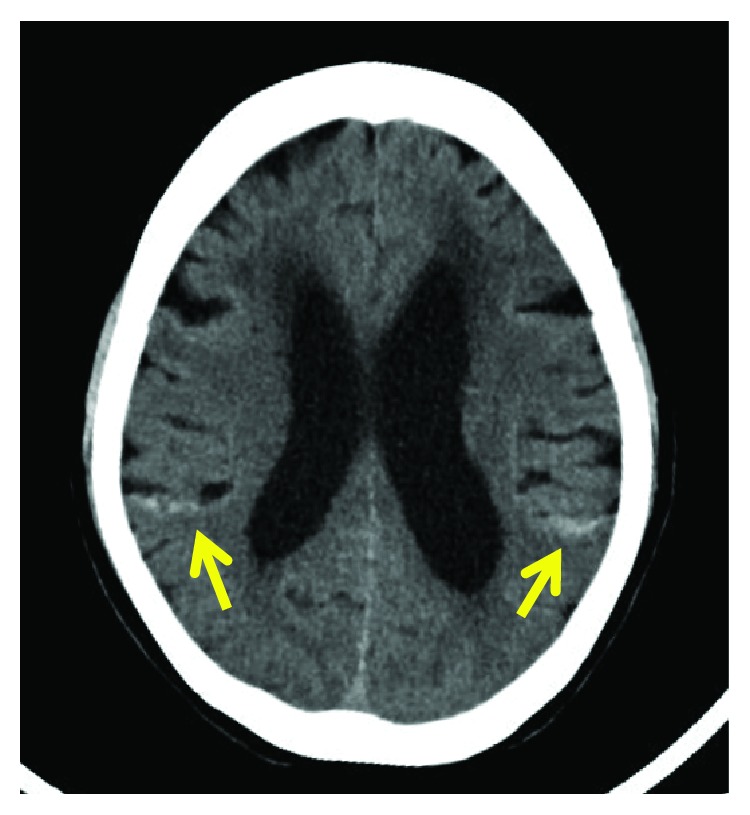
Axial noncontrast brain computed tomography showing a subarachnoid hemorrhage in the bilateral hemispheres at 7 days after onset.
